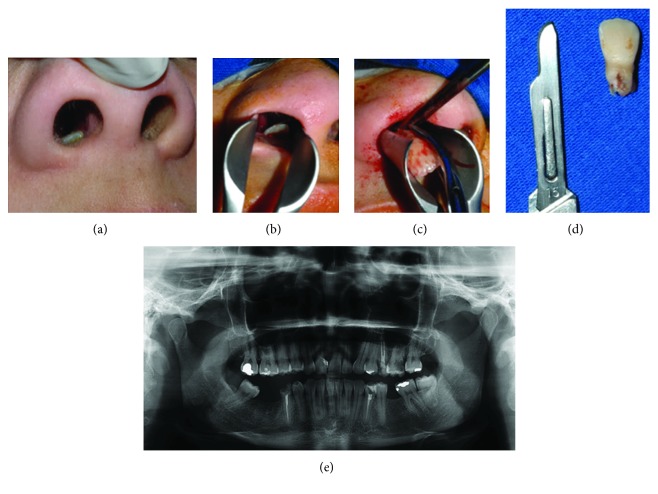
(a) Preoperative picture showing the exposition of the ectopic tooth inside the right nostril. (b, c) With the aid of nasal speculum, the tooth was removed by intranasal approach. (d) Tooth removed. (e) Panoramic radiograph confirms the complete removal of the element 11.
